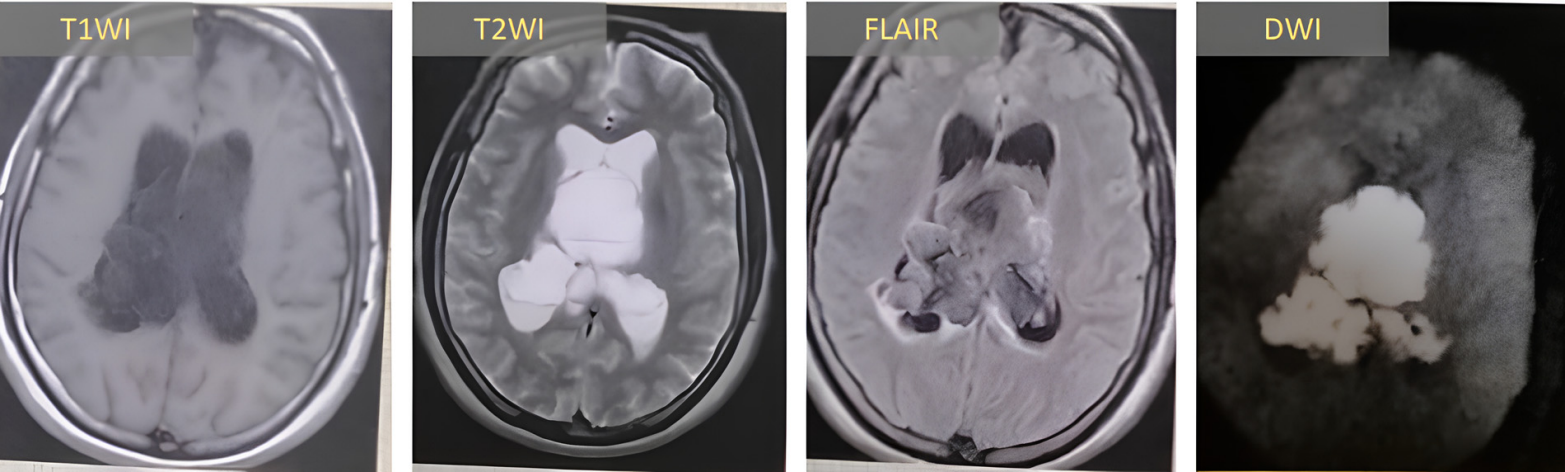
Preoperative brain MRI of a multiloculated intraventricular epidermoid cyst. The lesion displays: (A) hypointense signal on T1WI; (B) hyperintense signal on T2WI; (C) incomplete nulling on FLAIR; and (D) restricted diffusion on DWI. Note the mass effect with hypothalamic compression, suprasellar cistern effacement, and near-complete obliteration of the mammillo-pontine space. *T1WI: T1-weighted imaging; T2WI: T2-weighted imaging; FLAIR: fluid-attenuated inversion recovery; DWI: diffusion-weighted imaging.*
